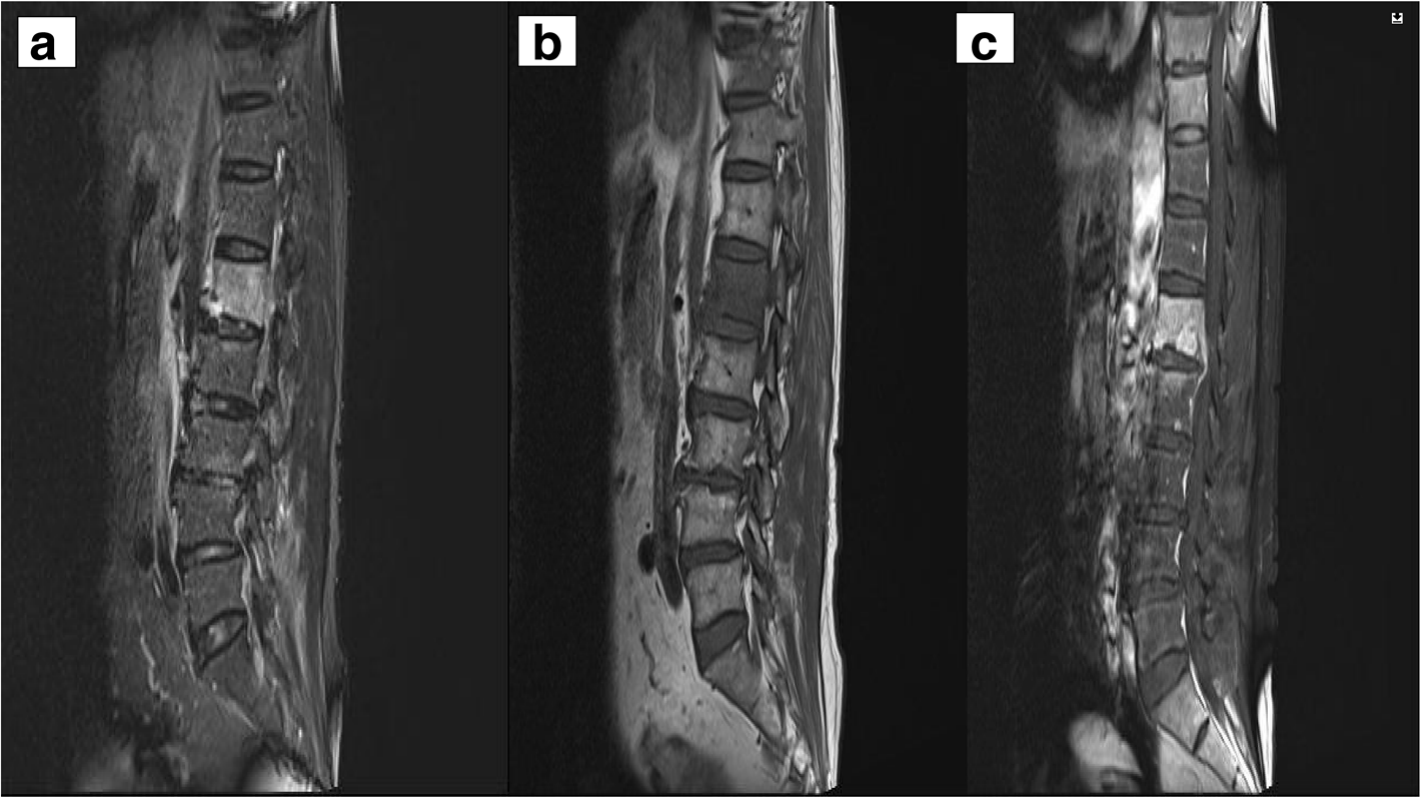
a L-spine T2_tse_sagittal, b L-spine T1_tse_sagittal, and c L-spine T1_tse_sagittal_fat suppressed. MRI of the lumbosacral spine shows altered signals in the L1 vertebral body (in the local clinic on 30 December 2017). MRI revealed whole bone marrow oedema with endplate lytic changes in the L1 body and focal marrow oedema in the upper endplate of L2 bodies. Additionally, it showed mild destruction of intervertebral disc at L1–2. Adjacent paravertebral soft tissue thickening and small abscess in left psoas muscle were found. These findings are considered to be indicative of pyogenic spondylitis
